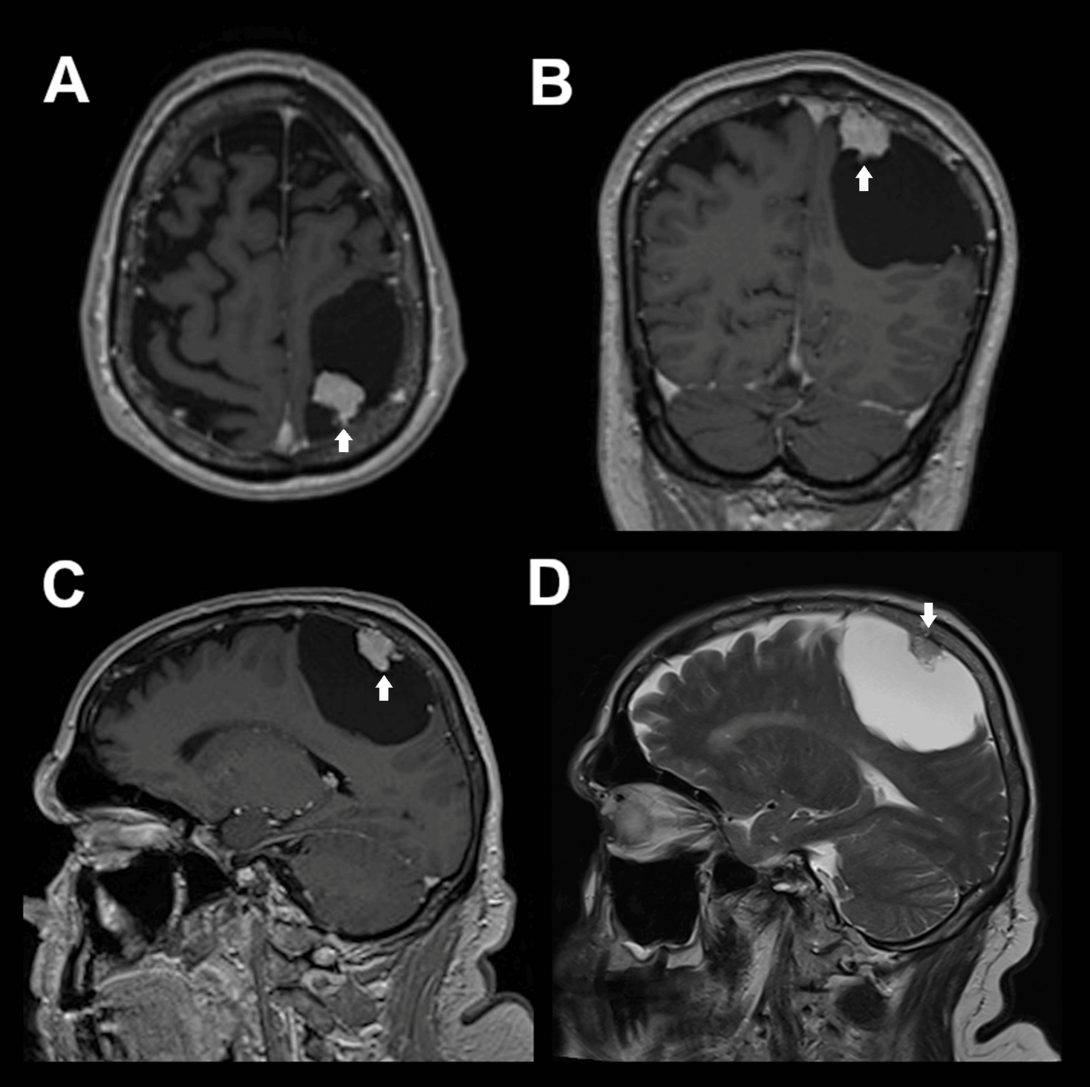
Preoperative MRI findings The images revealed a small contrast-enhancing lesion (white arrows) together with a large associated cyst in the paramedian left central region, suggestive of a hemangioblastoma (postcontrast T1 sequences in axial (A), coronal (B), and sagittal (C) views). T2 sequences did not show any signs of edema (D) MRI: magnetic resonance imaging
